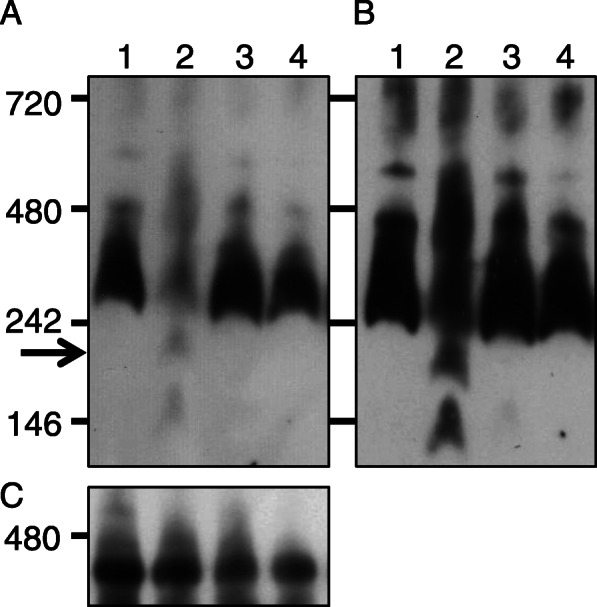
Immunoblot analysis of total cellular proteins from immature anthers collected from TA-33BB-CMS (lane 1), NK-198 (lane 2), TA-33BB-CMS x PI 615522 (lane 3) and TA-33BB-CMS x PI 518644 (lane 4). Protein samples were electrophoresed in Blue Native polyacrylamide gels (4–16%). Size markers (kDa) are shown on the left. An arrow indicates the 200-kDa band that is the hallmark of post-translational interaction with preSATP6. Immunoblots were probed with anti-preSATP6 (a and b) or anti-COXI (c). Exposure times to X-ray film were 10 s and 1 min for a and b, respectively
